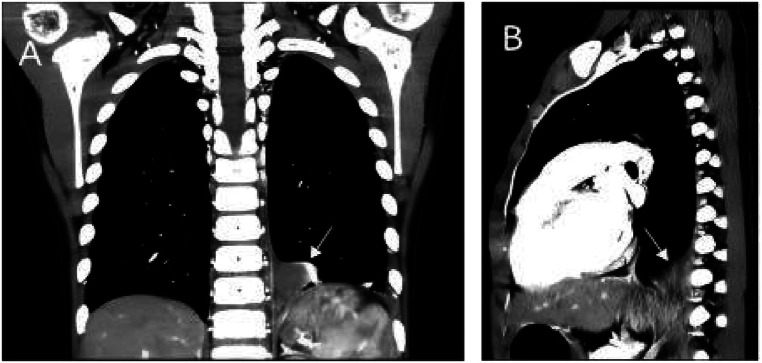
Contrast-enhanced chest CT. (A) A soft-tissue mass (arrow) is noted with peripheral enhancement along its margins. (B) A soft-tissue mass (arrow) is shown with no definite arterial feeding vessel identified.
